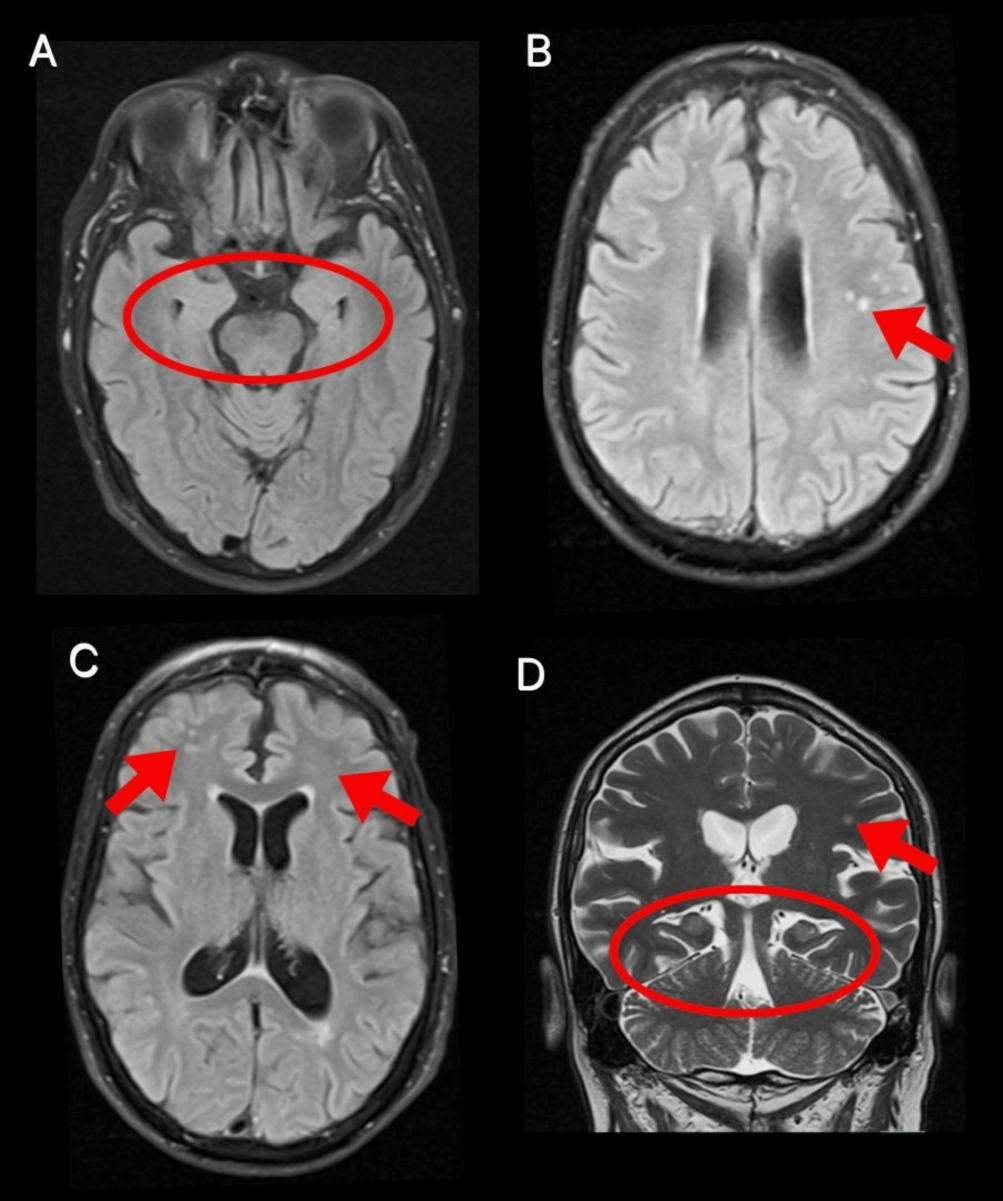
Brain MRI (A) FLAIR sequence, coronal view: hippocampi (red circle) appear symmetric with no signal abnormalities. (B) FLAIR sequence, axial view: multiple punctate subcortical hyperintensities in the left parietal region (red arrow). (C) FLAIR sequence, axial view: multiple punctate hyperintensities in the right frontal and periventricular regions (red arrows). (D) T2-weighted sequence, coronal view: nodular hyperintensity in the left parietal region (red arrow) with symmetric hippocampi (red circle). FLAIR: fluid-attenuated inversion recovery
